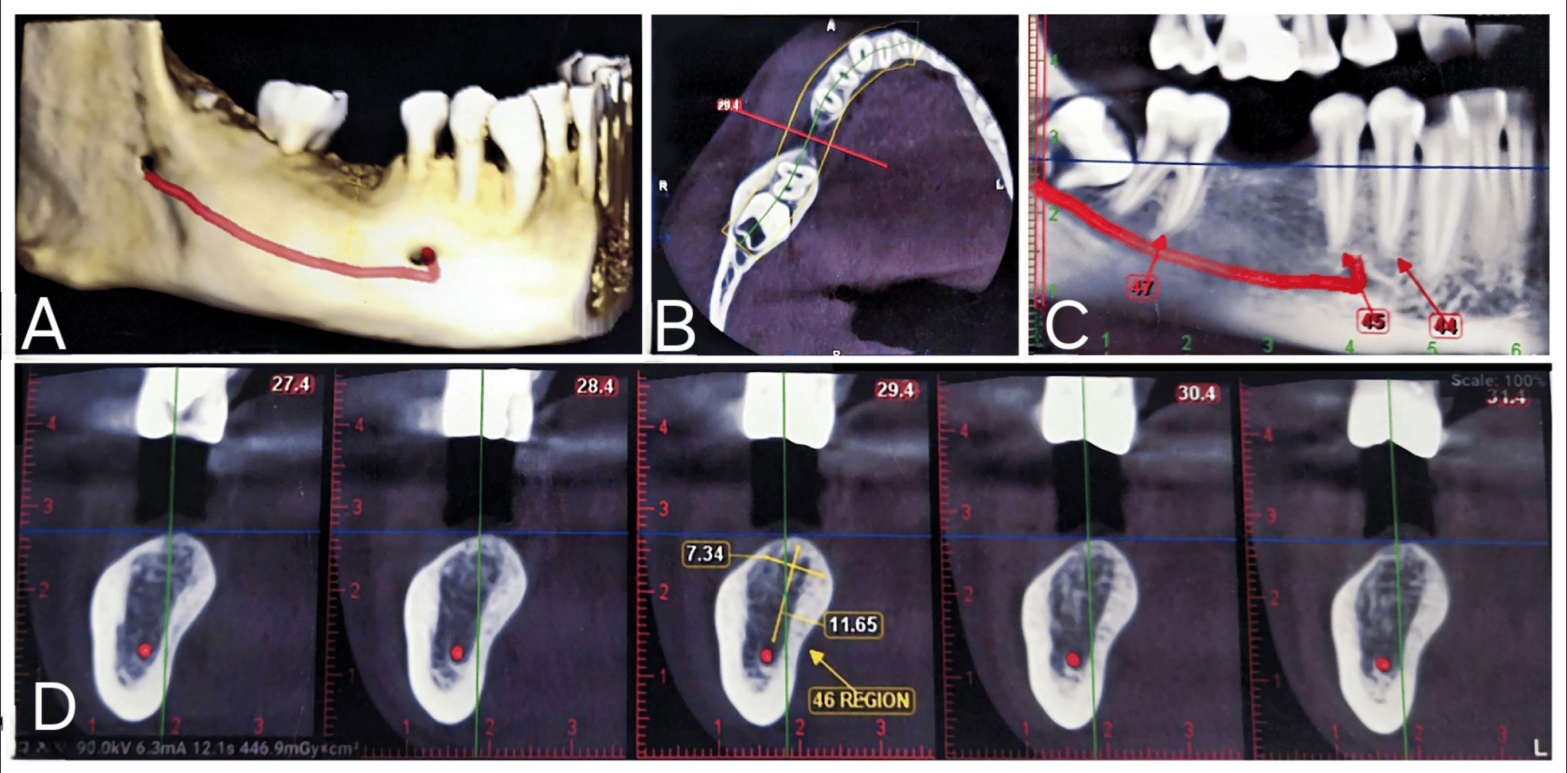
Detailed CBCT images of the mandibular region (A) Three-dimensional view of the mandible; (B) axial section of the mandible in the missing tooth region; (C) sagittal section of the mandible in the missing tooth region; (D) cut section of the missing tooth in the sagittal section, showing the dimensions of the bone CBCT: cone-beam computed tomography
